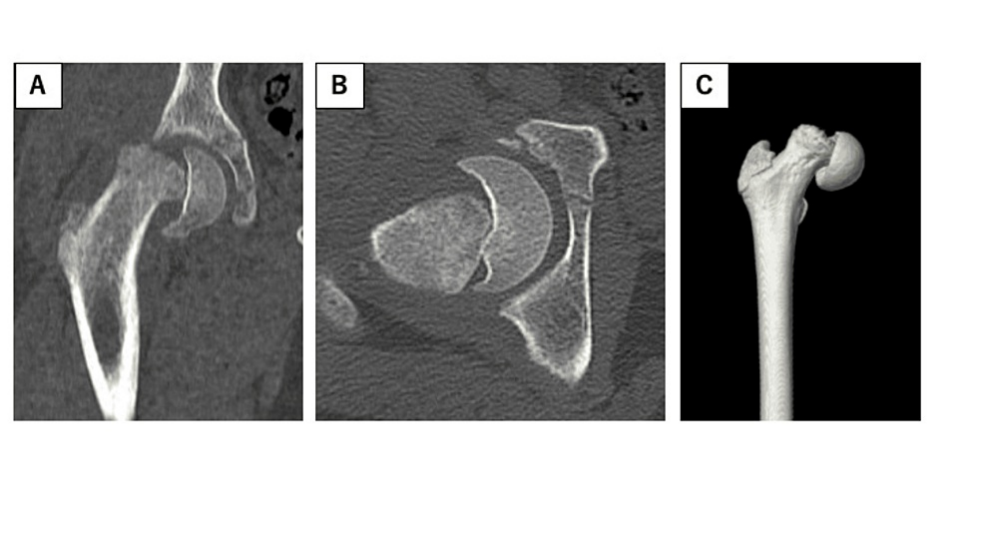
Computed tomography revealing 63° of internal rotation, with 15° of posterior dislocation relative to the unaffected side(arrow). (A) Coronal view; (B) axial view; (C) three-dimensional view.
